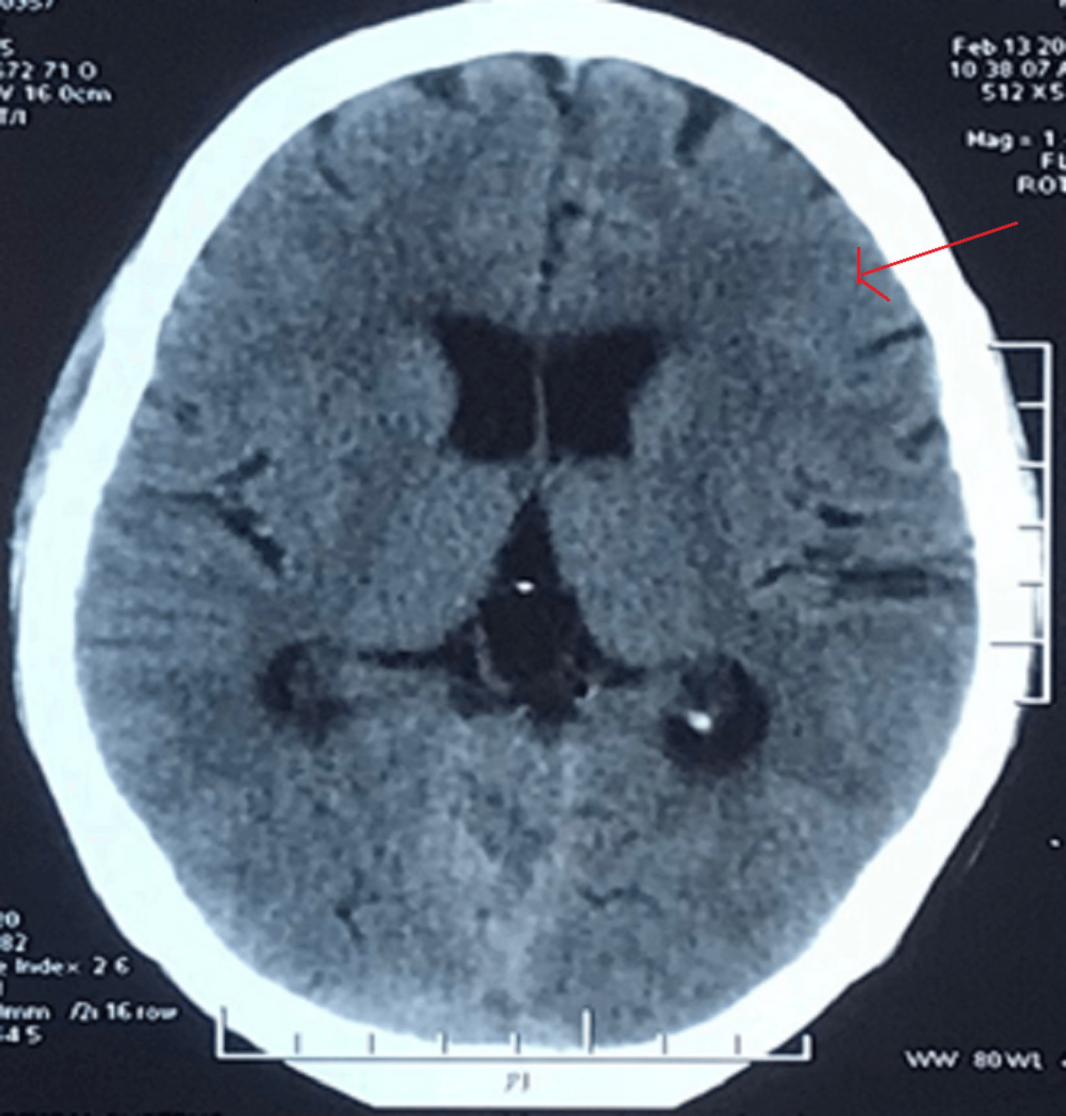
Pre-operative CT head at the frontotemporal level showing no subdural hematoma.
